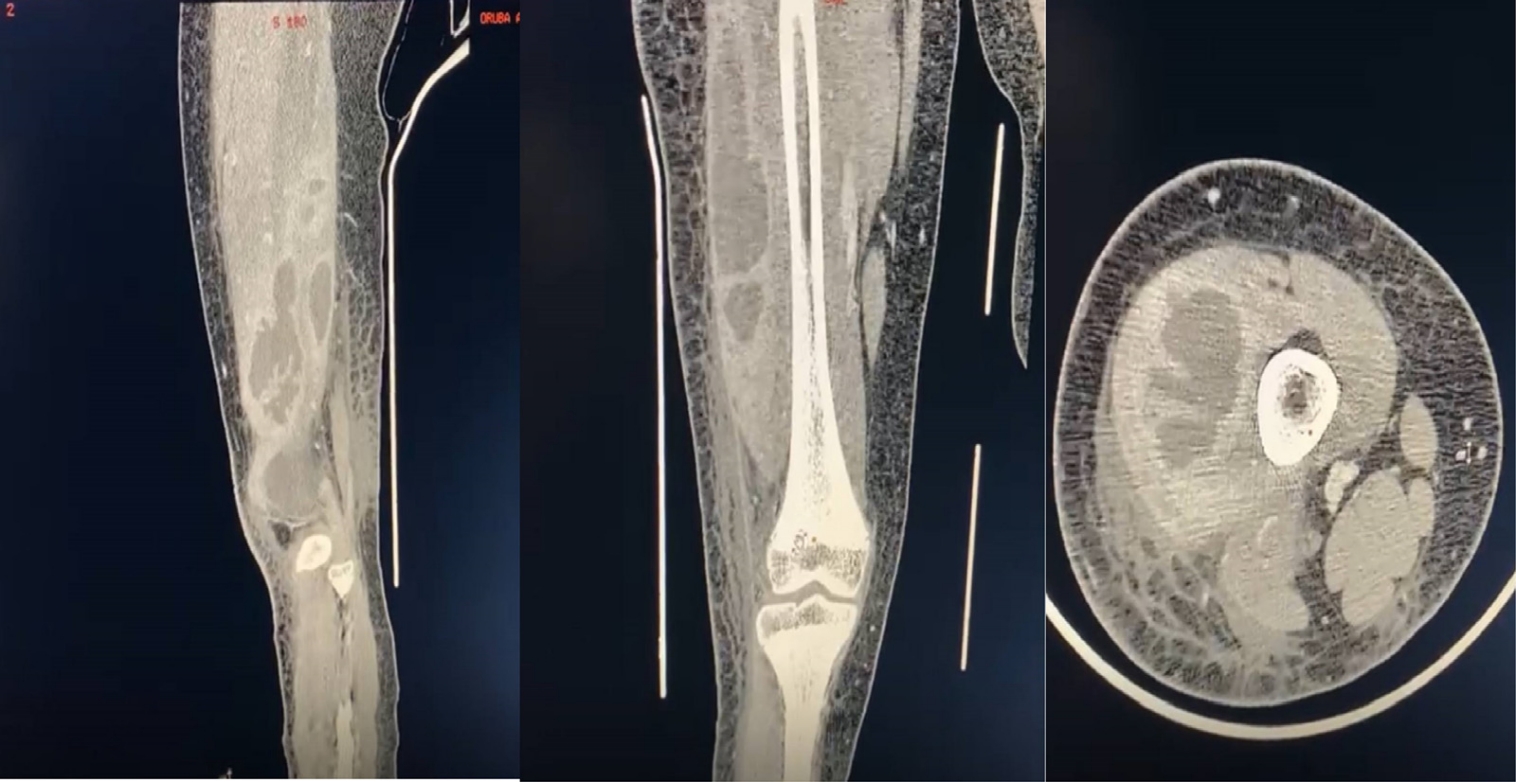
Sagittal, coronal and axial CT scan of Rt thigh showing intramuscular multilobulated collection.
